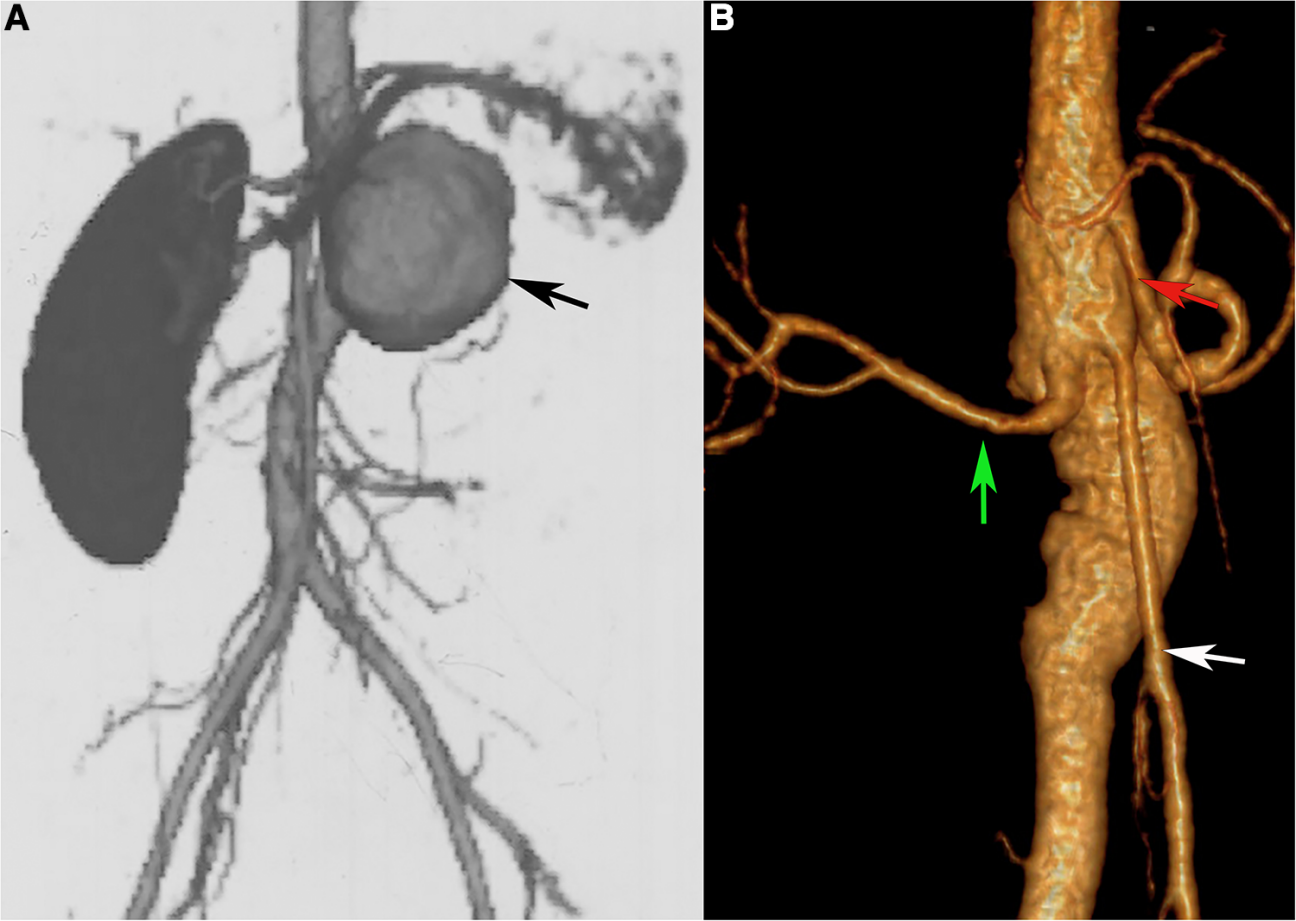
(A) abdominal aortic pseudoaneurysm (black arrow). (B) CT Angiography after pseudoaneurysm resection and abdominal aorta reconstruction. Green arrow, right renal artery. White arrow, superior mesenteric artery. Red arrow, celiac artery. Black arrow, abdominal aortic pseudoaneurysms.
